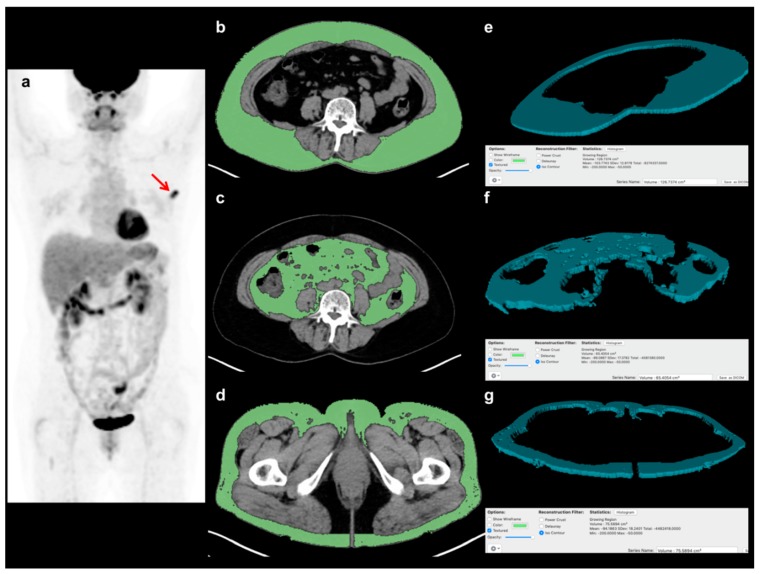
Example of the measurement of volume and CT-attenuation of visceral adipose tissue (VAT), abdominal subcutaneous adipose tissue (SAT), and gluteofemoral adipose tissue (AT). A 57-year-old woman with breast cancer underwent staging FDG PET/CT, showing focally increased FDG uptake at the left breast in a maximum intensity projection image (a) (arrow). Using three consecutive transaxial CT images of FDG PET/CT, the areas of VAT, abdominal SAT, and gluteofemoral AT were delineated using a CT-attenuation range of −200 to −50 HU at (b) the L4–5 spine level for VAT and (c) abdominal SAT, and (d) at the proximal femur level for gluteofemoral AT. Based on the areas of each AT in the CT images, (e) the three-dimensional structure of VAT, (f) abdominal SAT, and (g) gluteofemoral AT were automatically created, and their volume and CT-attenuation values were calculated.
